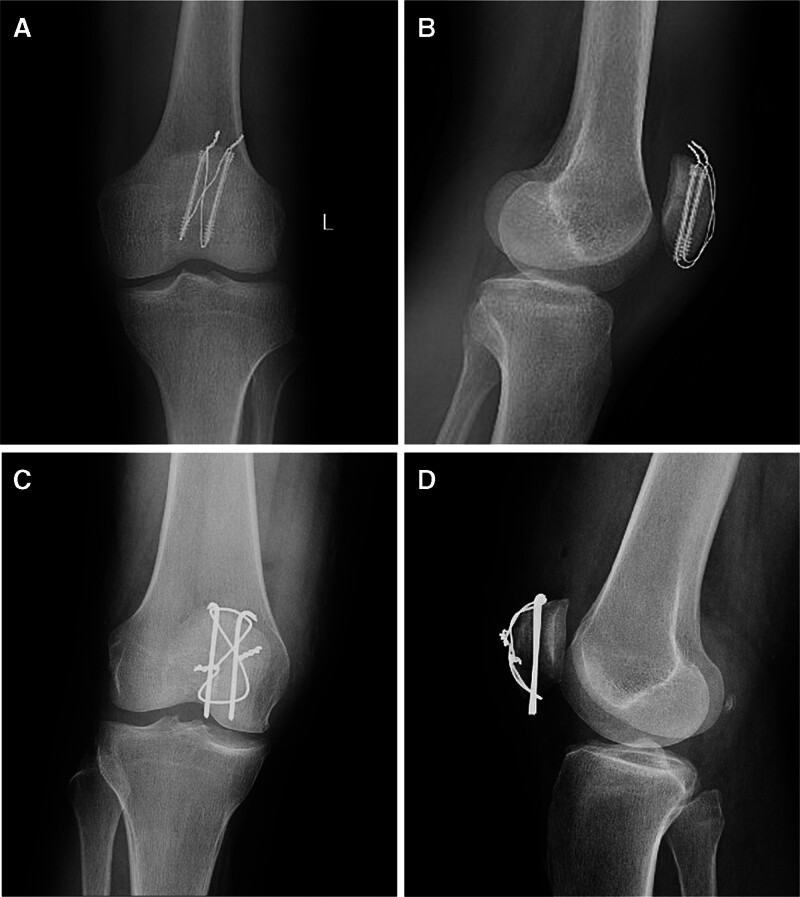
The 2-d postoperative radiographs of fracture reductions and implant positions. (A) Posteroanterior and (B) lateral X-rays of Patient 1 at 2 d post-operation with the MIOT technique; (C) posteroanterior and (D) lateral X-rays of patient 2 at 2 d post-operation with the open surgical technique.
